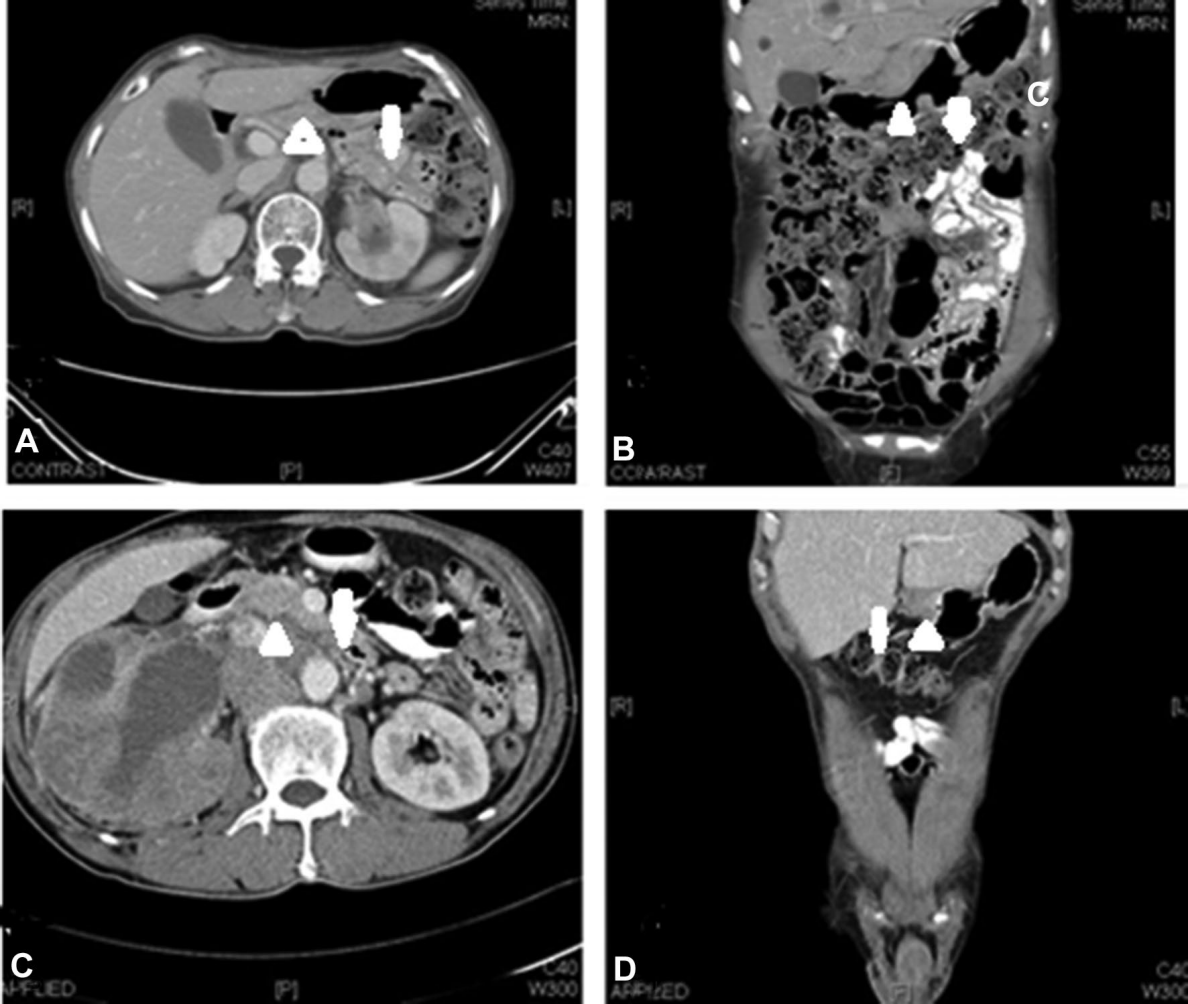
CT scans showing peritoneal thickening of renal pelvis carcinoma complicated by gastrointestinal bleeding. Case 1: a axial and b coronal views. Case 2: c axial and d coronal views. a–d All show thickened peritoneum and tumor invasion into gastrointestinal tract. Arrows indicate a thickened or dirty peritoneum. Arrowheads indicate tumor invasion into the gastrointestinal tract
